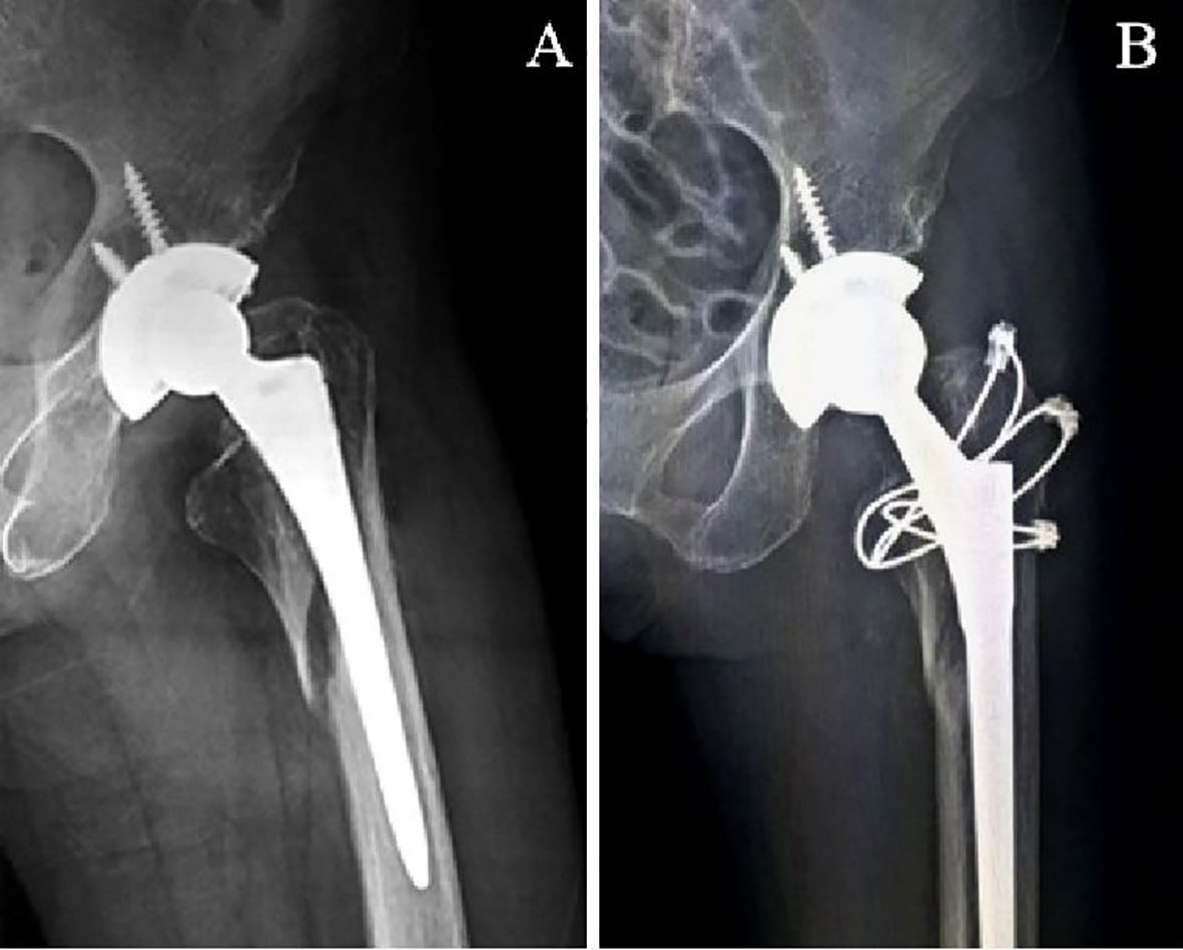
Case 2, a 60-year-old woman with a left femoral neck fracture due to a fall. (A) Preoperative lateral radiograph. (B) Postoperative anterior–posterior radiograph after longer stem revision and internal fixation with cables.
